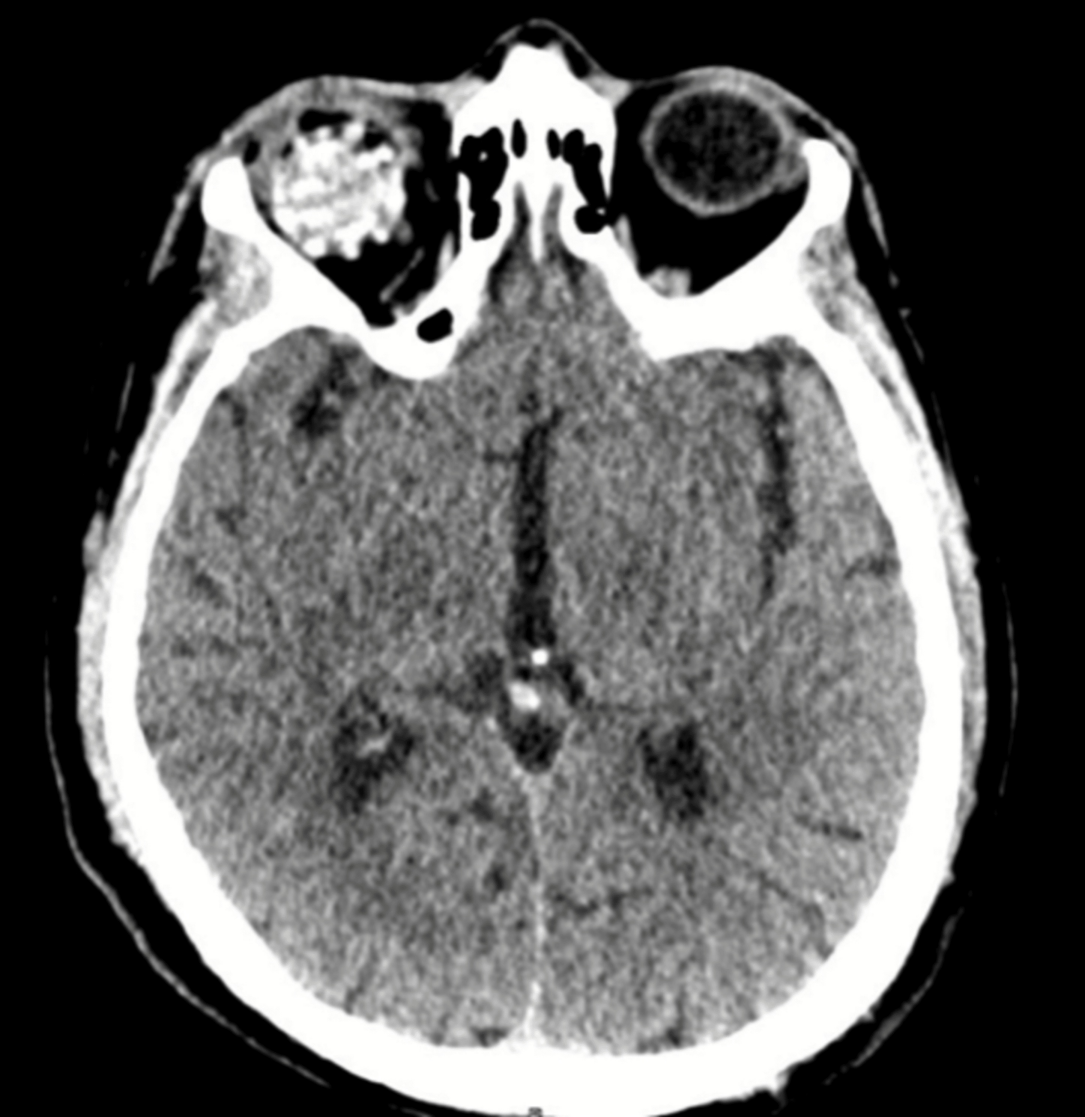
CT scan The axial section shows the hyperdense multi-celled implant. CT: computed tomography
